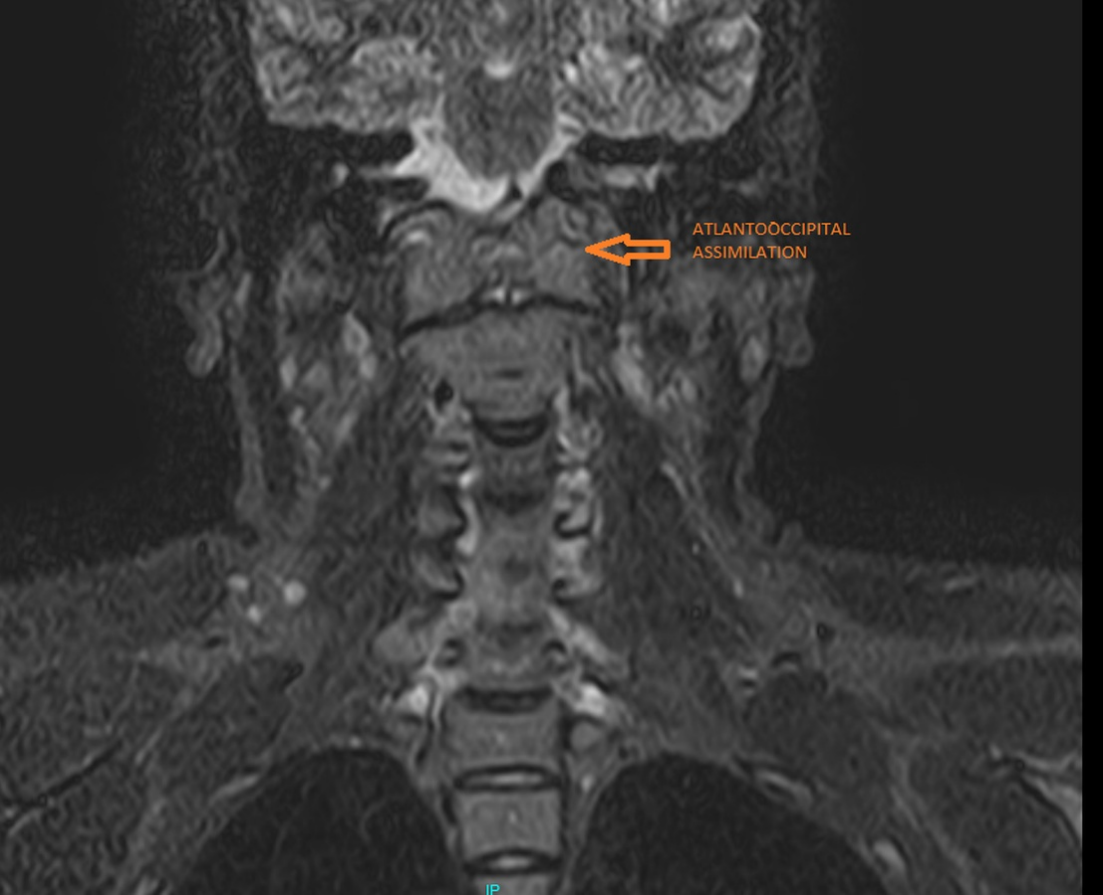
Coronal short tau inversion recovery (STIR) magnetic resonance imaging scan showing complete atlantooccipital assimilation (arrow)
